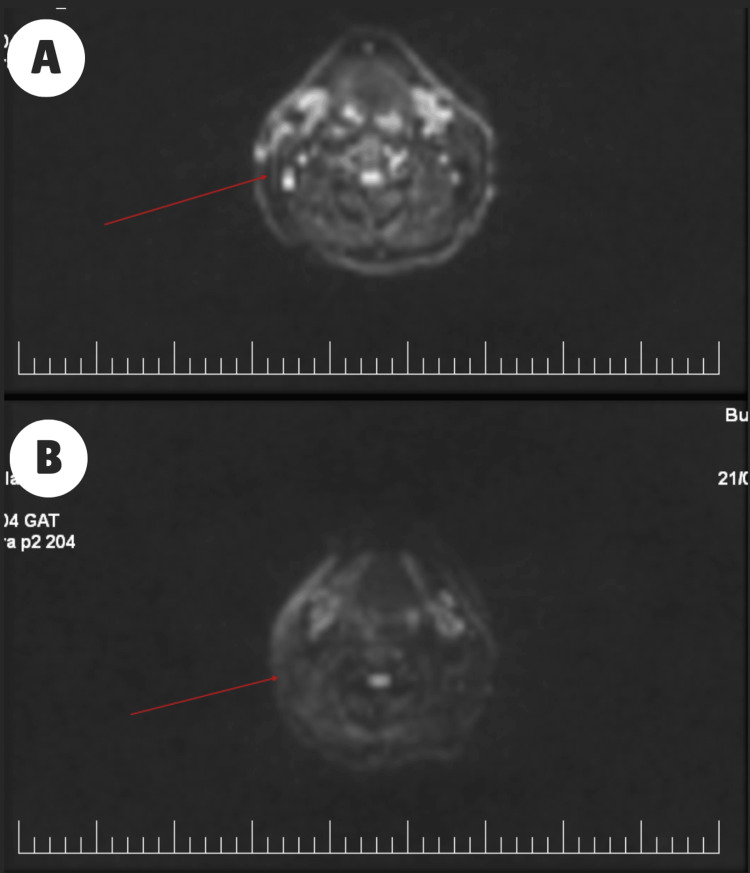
Diffusion MRI showing the evolution of the lymphadenopathies (marked with red arrow) before RT (A) and after RT (B). MRI: magnetic resonance imaging; RT: radiotherapy
